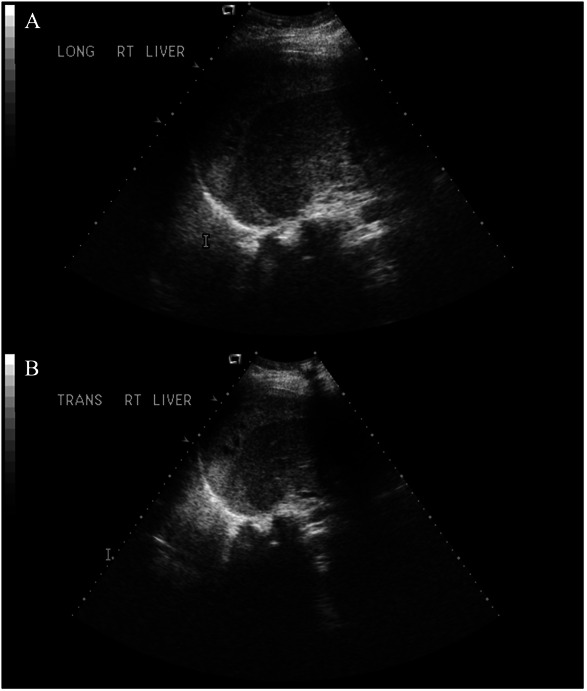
Longitudinal view (A) and transverse (B) ultrasound imaging of the liver of a patient with subcapsular hematoma.
